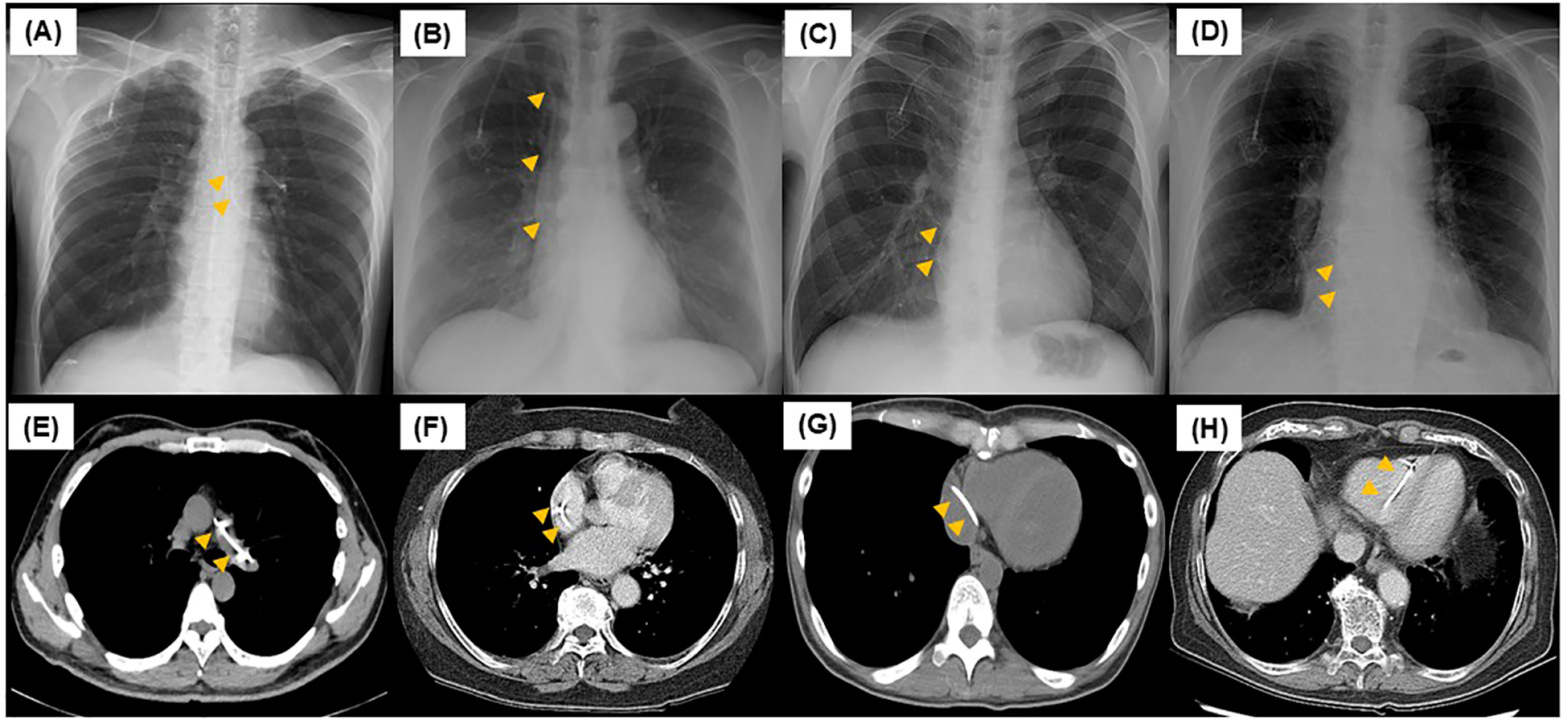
Chest radiography and computed tomography scan at central venous port rupture. For case 1 (A, E), the ruptured catheter was in the left pulmonary artery. For case 2 (B, F), the ruptured catheter was in the superior vena cava. For cases 3 (C, G) and 4 (D, H), the ruptured catheter was in the right atrium.
